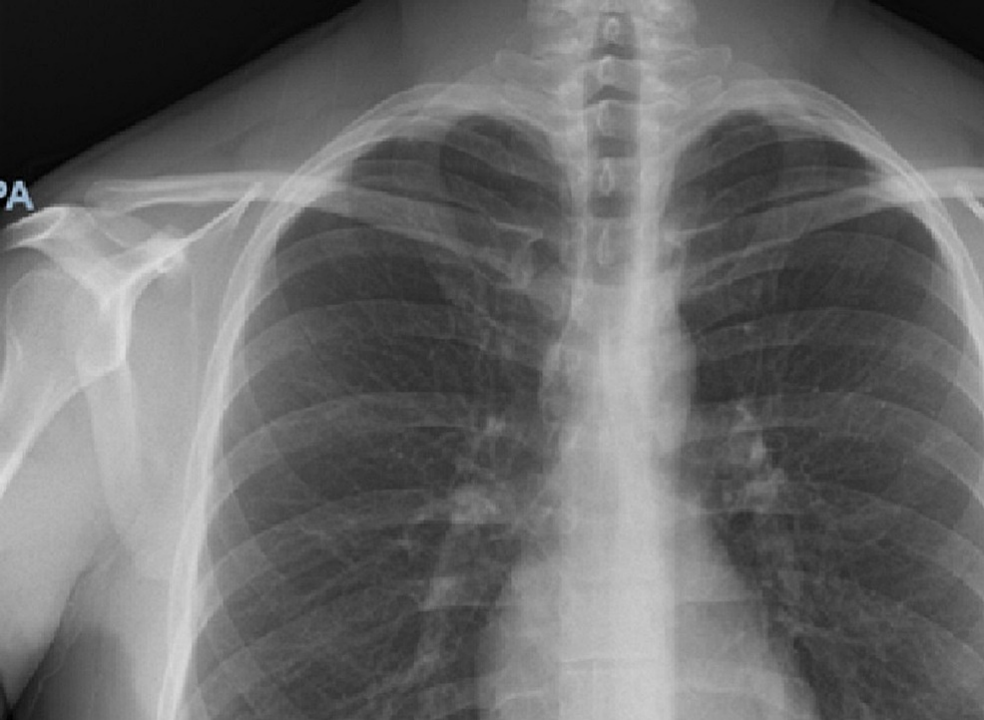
Chest x-ray on admission. There is no focal consolidation or congestive heart failure. There is no pleural effusion, cardio-mediastinal silhouette is not enlarged, and trachea is in the midline. There is no pneumothorax.
